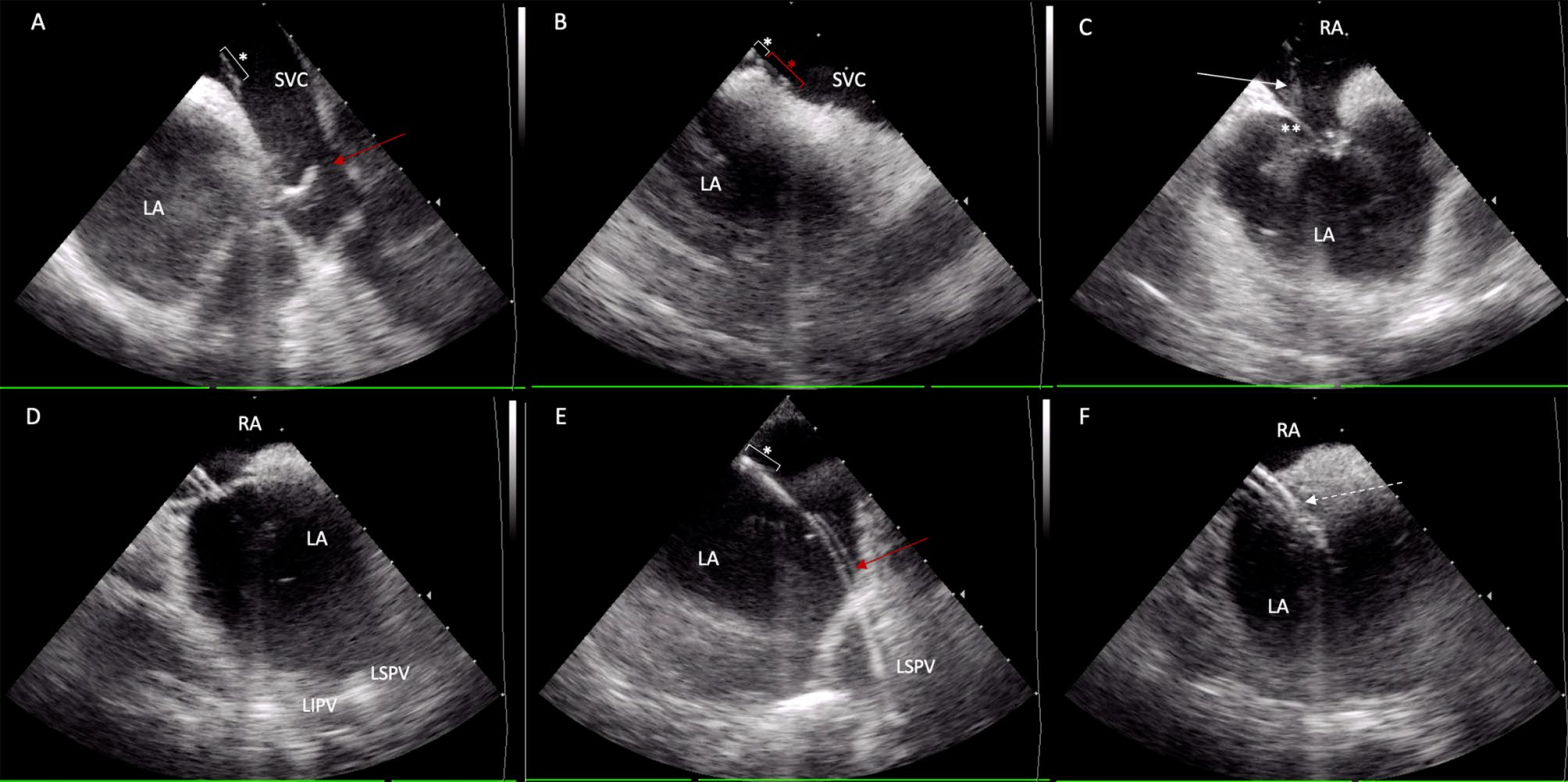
Llustrative intracardiac echocardiography (ICE) images capturing crucial moments in the zero-fluoroscopic transseptal puncture procedure. Panel (A) shows the guidewire (red arrow) and the fixed-cure long sheath (white asterisk) located in the superior vein cava. In Panel (B) the guidewire is switched to a transseptal needle (red asterisk) visible at the end of the fixed sheath (white asterisk), both still located in the SVC. Panel (C)—by pulling the sheath and the needle down (white arrow), they appear in the fossa ovalis (double white asterisk) creating the ”tenting” phase of the transseptal puncture. After transseptal puncture, in Panel (D) the sheath is visualized, inserted into the LA. Panel (E) shows the guidewire introduced in the LSPV, thus in the next step the fixed sheath can be replaced to a steerable sheath (dashed arrow), as in Panel (F). ICE, intracardiac echocardiography; SVC, superior vena cava; LA, left atrium; RA, right atrium; LSPV, left superior pulmonary vein; LIPV, left inferior pulmonary vein.
